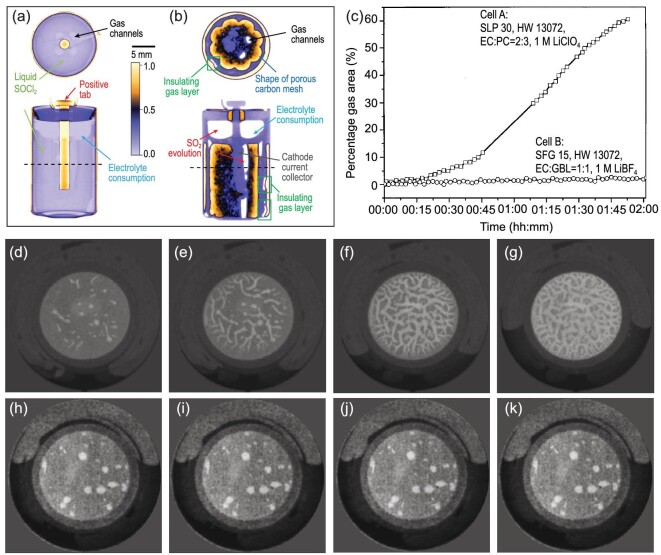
Application of NI in observing the gas generation in cells. (a and b) Horizontal and vertical orthogonal slices from the X-ray CT and neutron CT of the fully discharged Li/SOCl_2_ cells at 25 mA. Reprinted with permission from ref [59]. Copyright 2020, Institute of Physics. (c) Evolution of the percentage of the electrode area covered with gas bubbles for a propylene carbonate– (PC) based cell and a γ-butyrolactone- (GBL) based cell; (d–g) neutron images of gas evolution for a PC-based cell at different SoCs; (h–k) neutron images of gas evolution for a GBL-based cell at different SoCs. Reprinted with permission from ref [29]. Copyright 2004, Elsevier.
